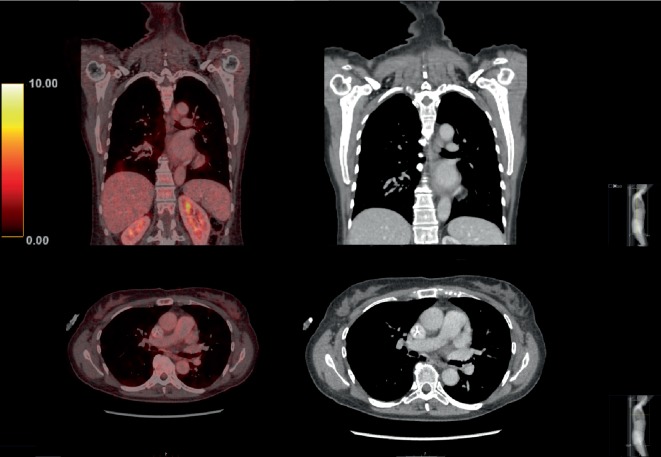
PET-CT images showing a complete radiological response of the lung metastasis. PET-CT (left column) and CT (right column) images.
